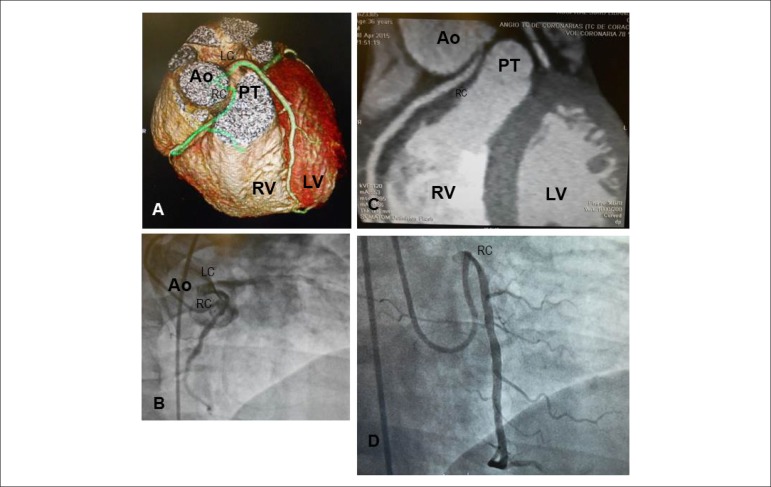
Similar projections of coronary arteries by computed tomography
